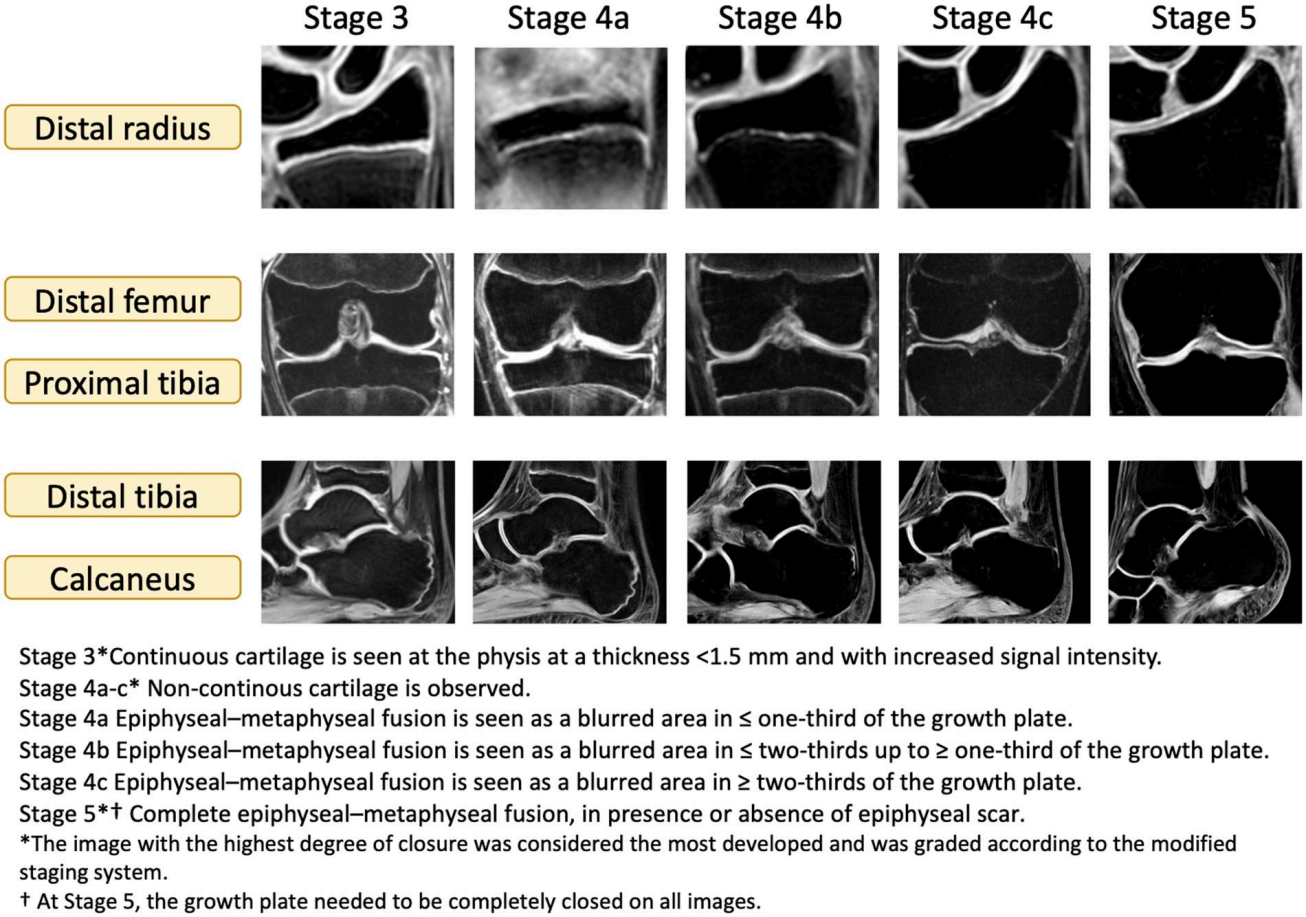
MRI images with the related MRI grading system based on the modified version of Kellinghaus’^17^ and Dedouit et al.^11^
